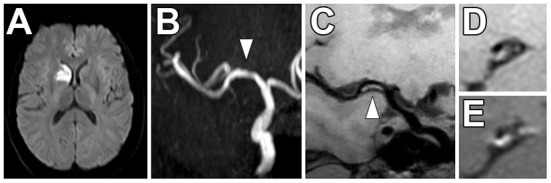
Non-stenotic right middle cerebral artery enhancing culprit plaque. (A) A patient with a right basal ganglia acute infarct showed (B) mild luminal irregularity but no appreciable stenosis of the right middle cerebral artery on time-of-flight MRA imaging (arrowhead). (C) Precontrast VW-MRI showed eccentric wall thickening along the right M1 middle cerebral artery (arrowhead). (D) Precontrast and (E) postcontrast images in the orthogonal plane through the plaque shows eccentric wall thickening and enhancement of the culprit plaque, which likely caused the ischemic infarct.
